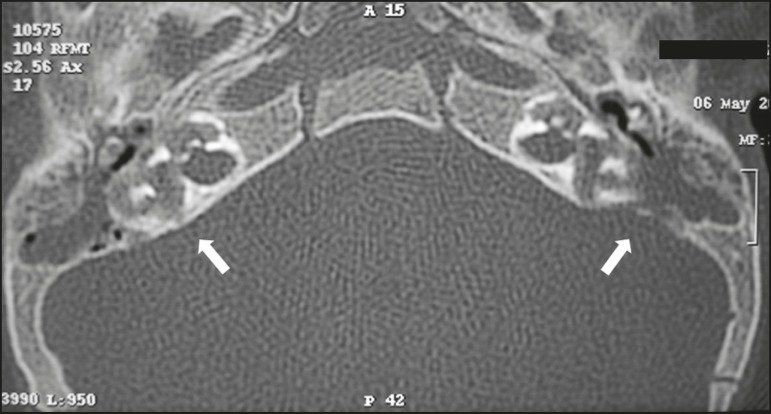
Otomastoiditis in an immunocompromised patient. Axial CT, with a
